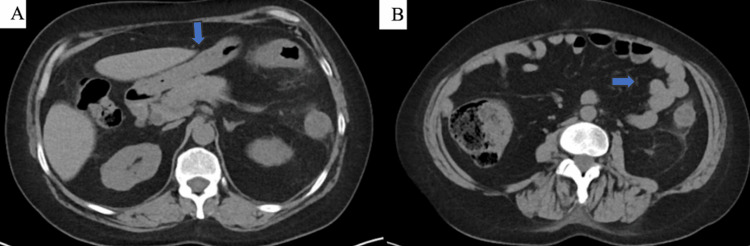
CT abdomen and pelvis with IV and oral contrast Colonic wall thickening and stranding involving the transverse colon (A) and descending colon (B).
